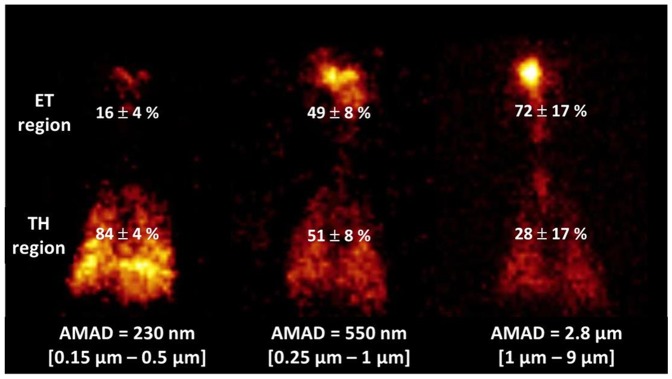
In vivo* inhalation experiments using baboons. Representative scintigraphic images obtained for the three studied aerosols. All images are from the same baboon. Total aerosol depositions (%) for the extrathoracic (ET) and thoracic (TH) regions are indicated. Activity median aerodynamic diameter (AMAD) and [d16, d84] were noticed for each aerosol generated.
